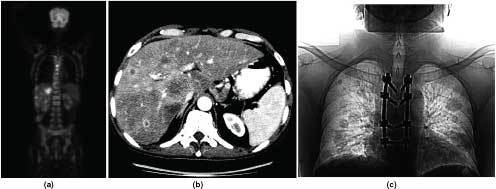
(a) Coronal positron emission tomography scan slice revealing highly avid lesion in the liver, thoracic vertebrae as well as less avid lesions in the lungs; (b) multiple lesions in the liver on CT scan; (c) post-vertebral fixation image revealing multiple cannonball metastases in both lungs.
